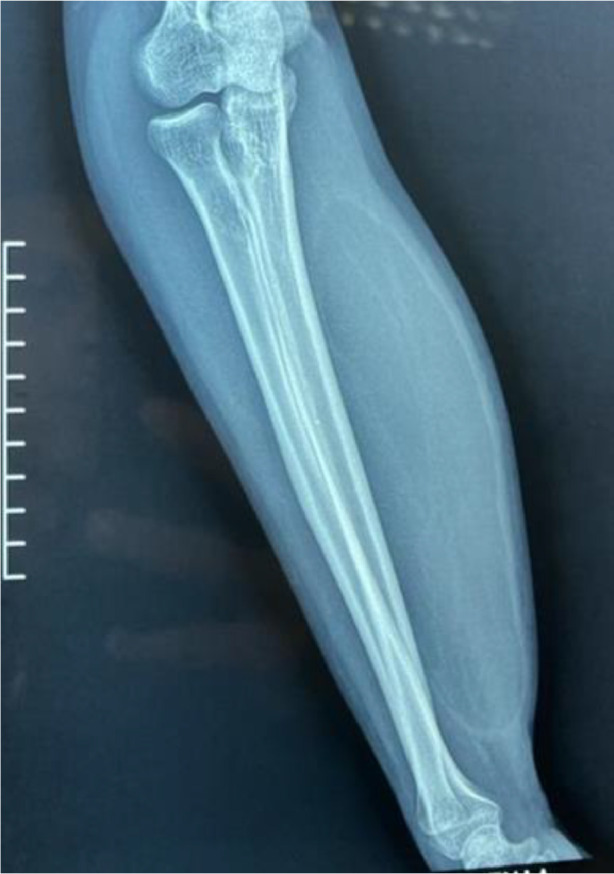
Standard X-ray of the forearm showing no anomalies.
